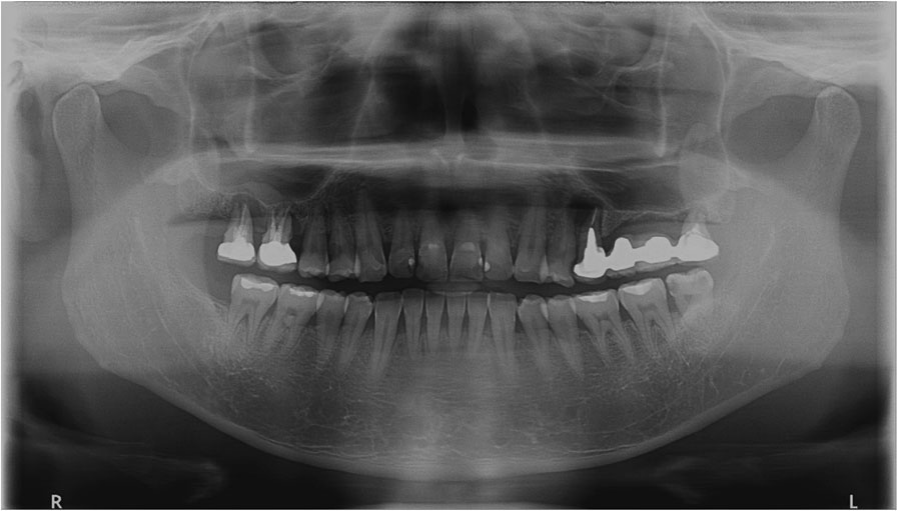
Panoramic radiograph: vertical alveolar bone loss and periapical abscesses were observed in the right maxillary first and second molars, the left maxillary second premolar, and the third molar. The sites were treated with root canal therapy
